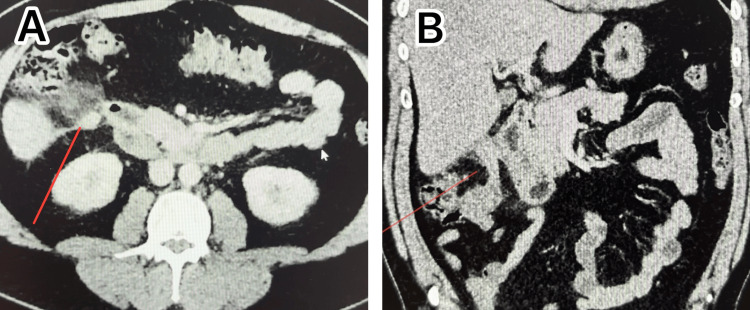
CT findings showing (A) the axial view with the red line pointing towards the appendiceal inflammation and (B) coronal view with red line pointing towards subhepatic appendix
